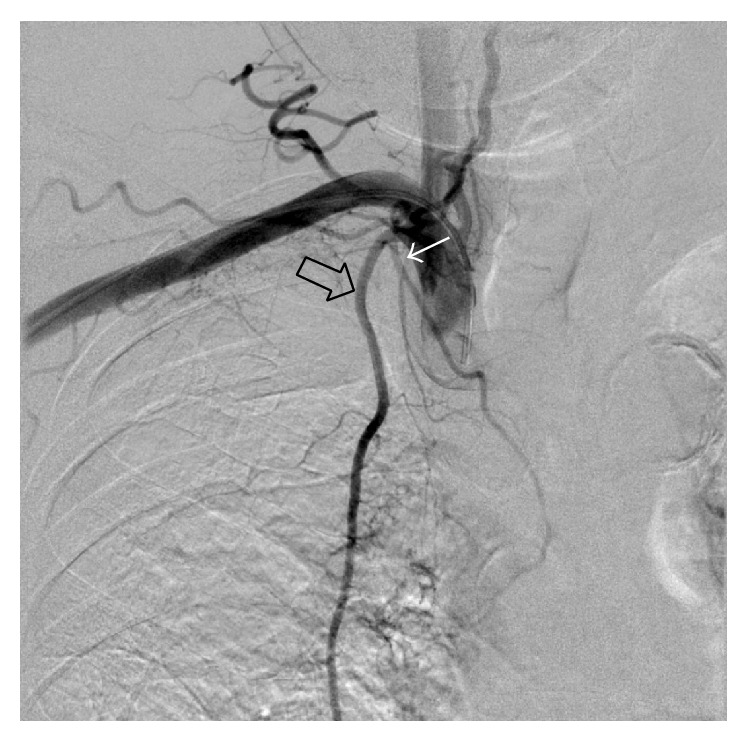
Angiogram of the brachiocephalic artery shows the hypertrophic aberrant bronchial artery (white arrow) arising from the proximal portion of the right internal mammary artery (black arrow), findings that corresponded with the CTA image.
